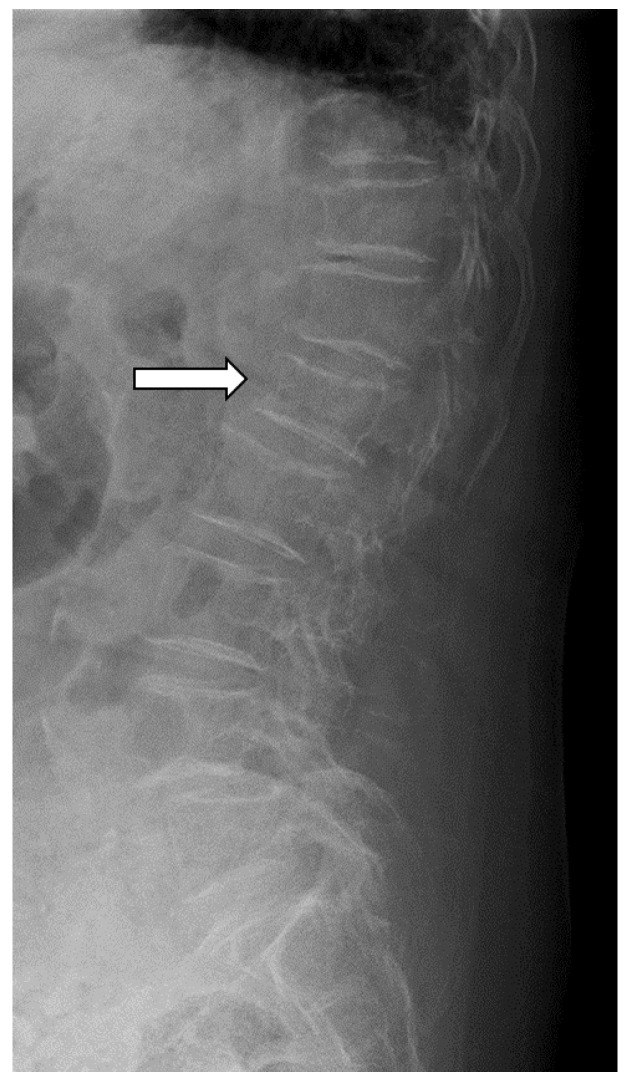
A 52-year-old female participant with L1 asymptomatic vertebral fractures (SQ grade 1). White arrows indicate mild compression of the first lumbar vertebra on the lateral lumbar spine X-ray image.
